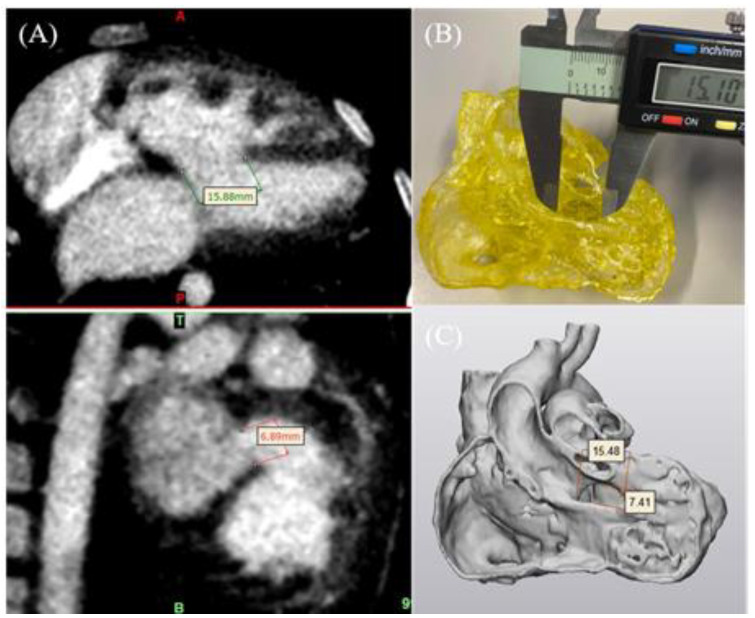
3D-printed model accuracy. (A) CT imaging data in coronal and sagittal views (left top and bottom images) to measure the VSD on source imaging data. (B) Measurement of the VSD in the 3D-printed model using a digital calliper. (C) STL file measurement of the VSD in 3-Matic. VSD—ventricular septal defect. Reprinted with permission under the open access from Lee et al. [22].
